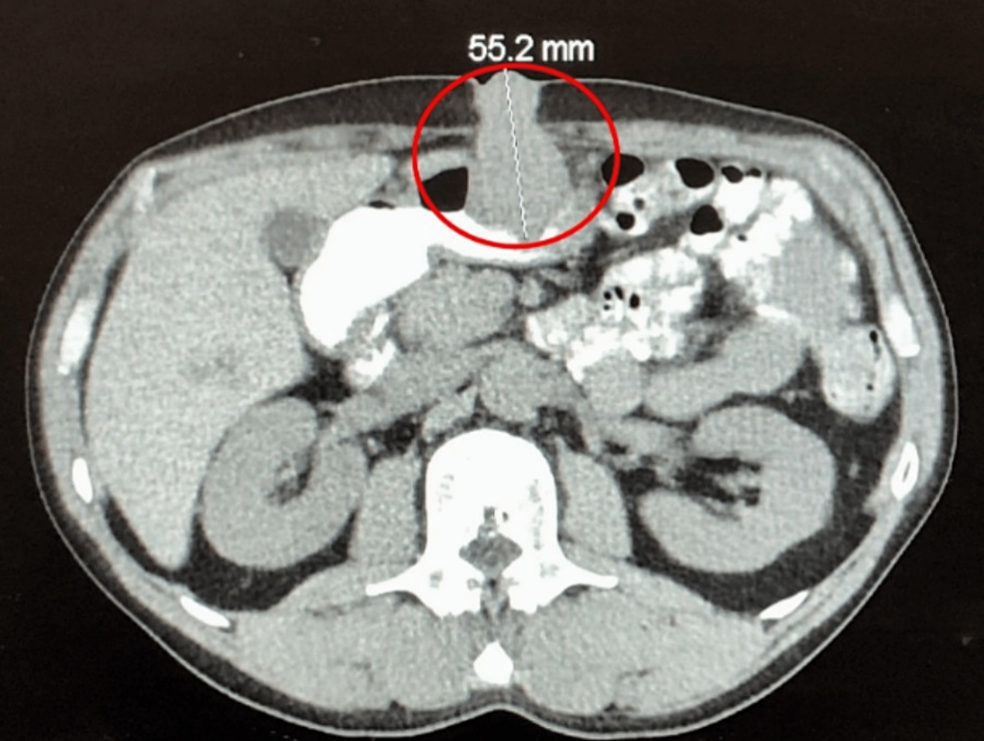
Computed tomography of the abdomen depicted a soft density (red circle) suggestive of a 5cm desmoid-like tumor at the percutaneous endoscopic gastrostomy site that extends onto the distal anterior surface of the antrum. No evidence of obstruction, free fluid, or pneumoperitoneum, and the appendix was unremarkable.
